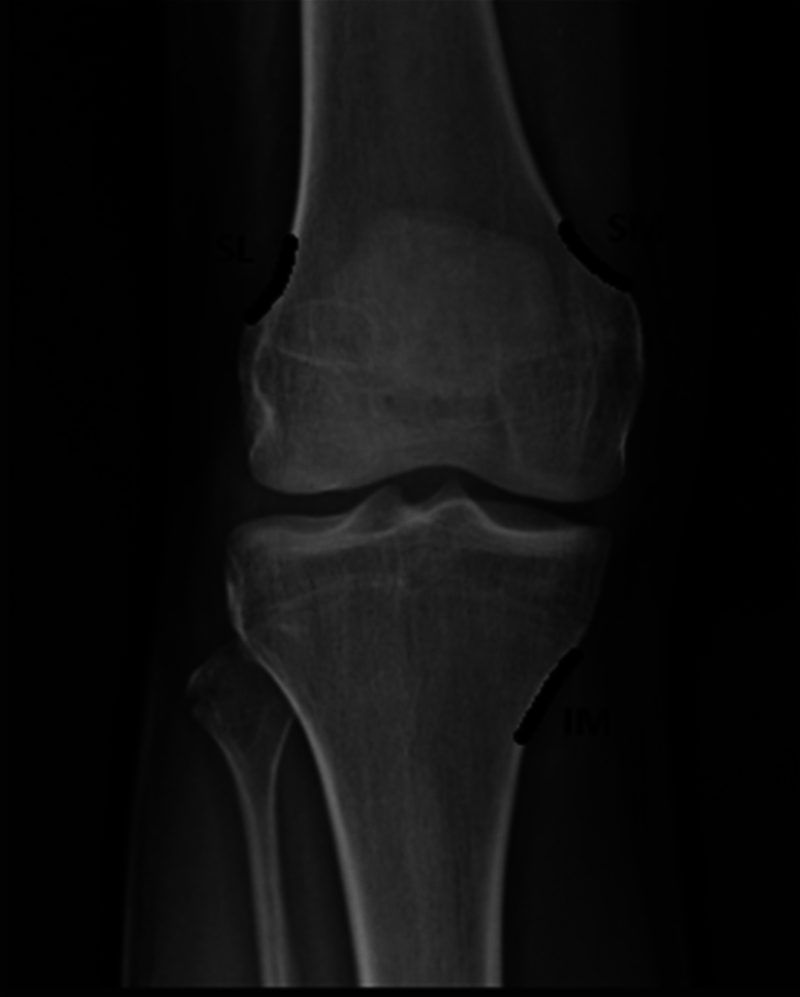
Anteroposterior radiograph demonstrating the reference points at fluoroscopy.
